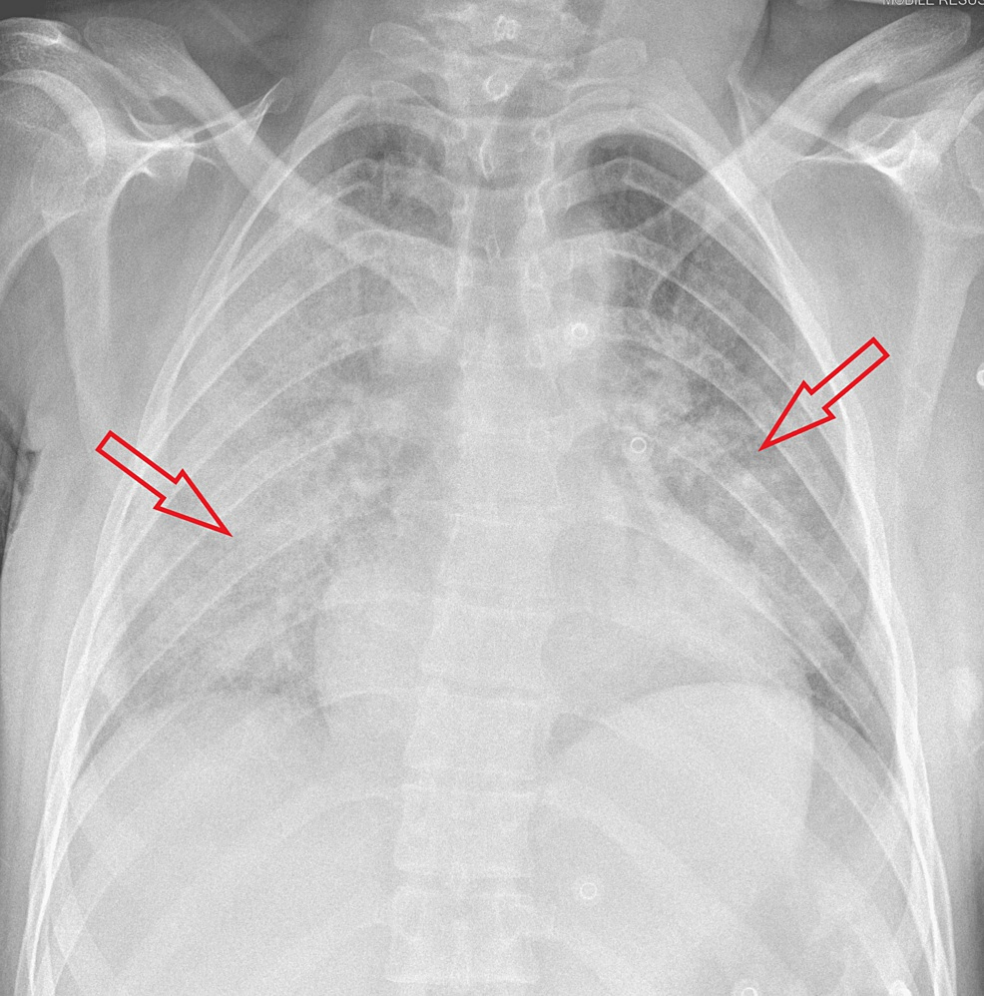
Chest X-ray from a previous admission showing bilateral infiltrates.
